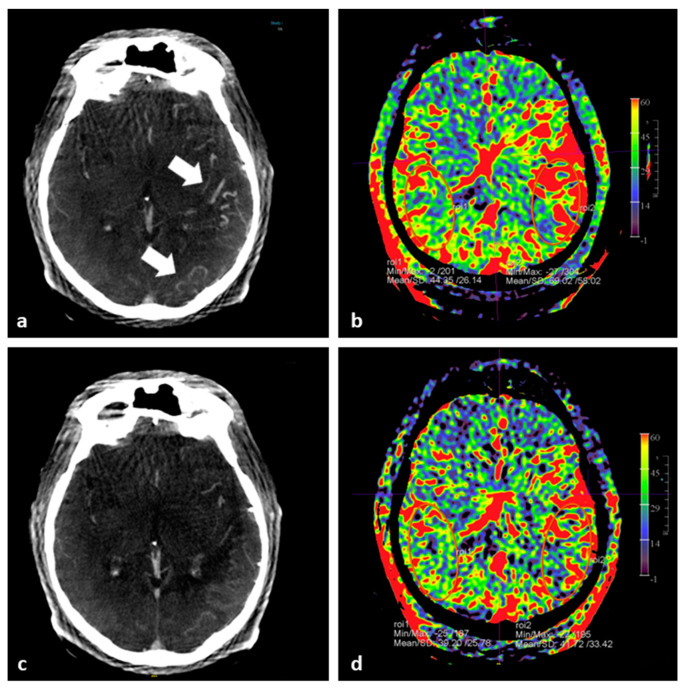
FD CT PBV imaging in DAVF treatment. (a) Axial FD CT fill run MIP reformation before treatment shows clearly the dilated and congested cortical cerebral veins at the right (white arrows). (b) Corresponding axial pretreatment PBV MIP reformation demonstrates elevated PBV values compared to the right side. (c) Axial fill run MIP reformation after partial embolization exhibits a clear diminishing of the cerebral venous congestion. (d) Post embolization axial PBV image shows symmetrical PBV values. The situation was accepted for the time being and the patient underwent uneventful a second embolization session 6 weeks later with complete occlusion of the DAVF.
